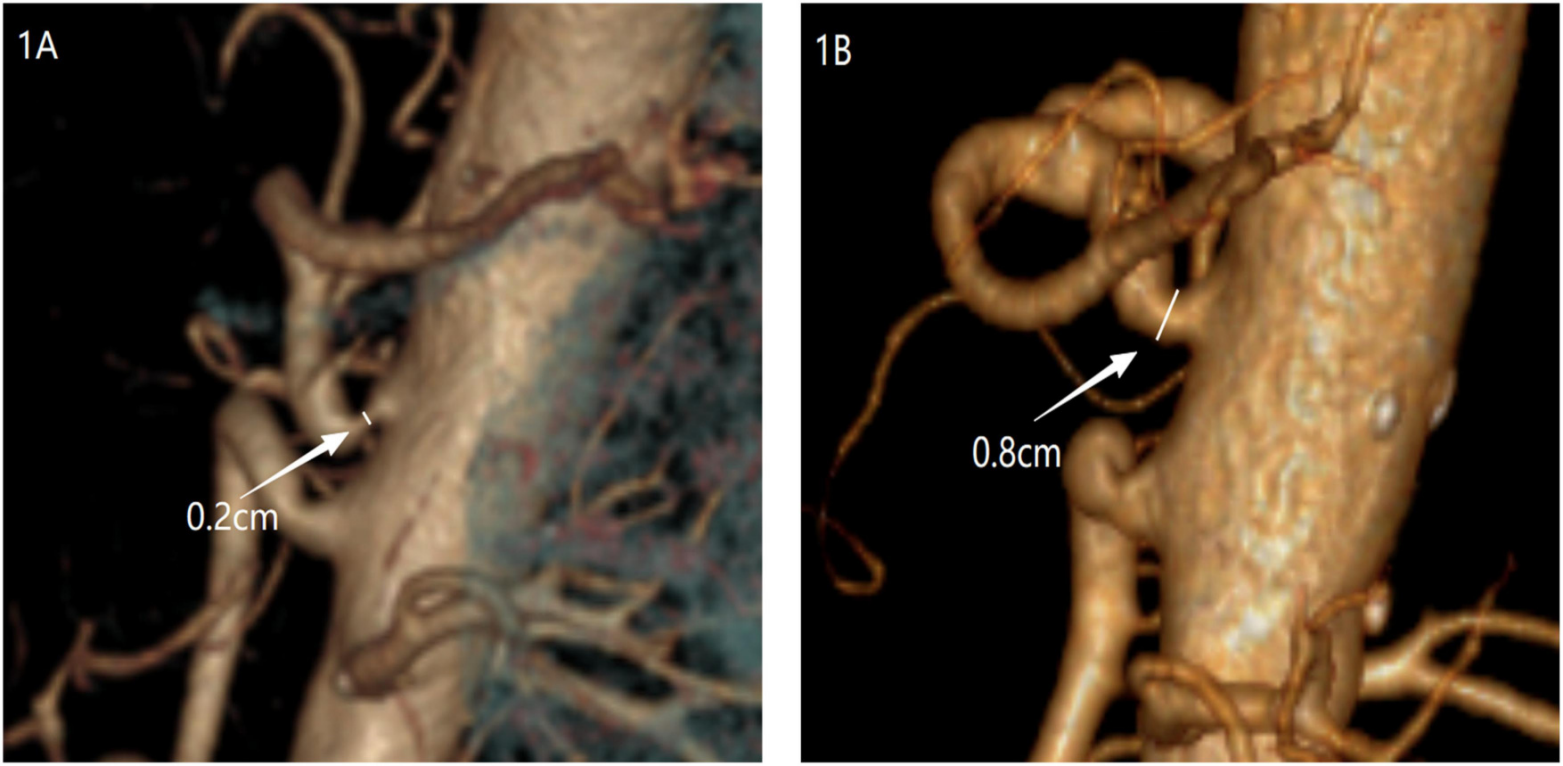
(A) CTA reveals a V-shaped narrowing at the origin of the CA with distal dilation, with the narrowest diameter measuring approximately 0.2 cm. (B) CTA shows that the origin of the CA is markedly dilated compared with prior imaging, with the narrowest segment measuring approximately 0.8 cm.
